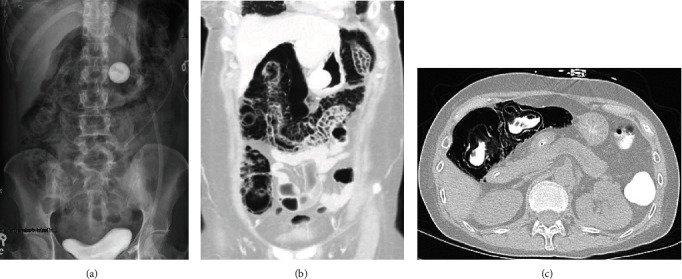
(a) Abdominal radiograph, (b) coronal CT, and (c) axial CT. PI of the ascending and transverse colon with “bubbly/expansile” wall appearance with extensive air cysts in the subserosal and submucosal layers.
